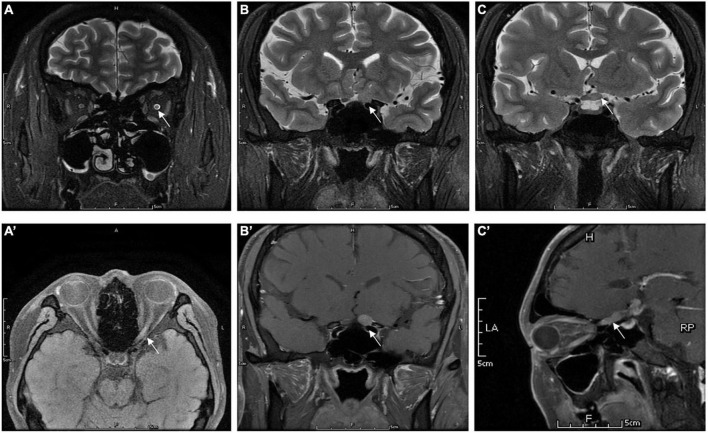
Orbital MRI images in patients who were diagnosed with IGM (case 7): panels (A) (axial), and (B,C) (coronal) showed the hypersignal of the optic nerve (the white arrow) extends to optic chiasm in T2WI. Panels (A′) (axial), (B′) (coronal), and (C′) (sagittal) showed the enhancement of left ON (the white arrow) in gadolinium-enhanced T1.
